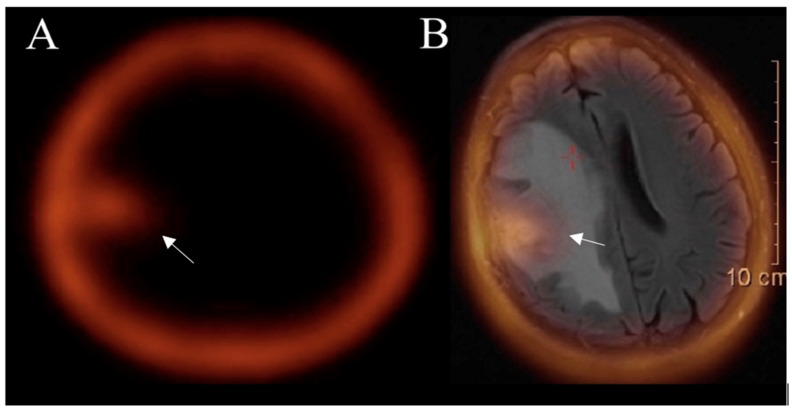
SPECT (A) and SPECT-MRI-fused (B) images show an accumulation of 99mTc- MIBI in a tumor area (arrow) after treatment, confirming the true progression of glioblastoma. Images used for publication are taken from LUHS Radiology Clinic archives servers.
